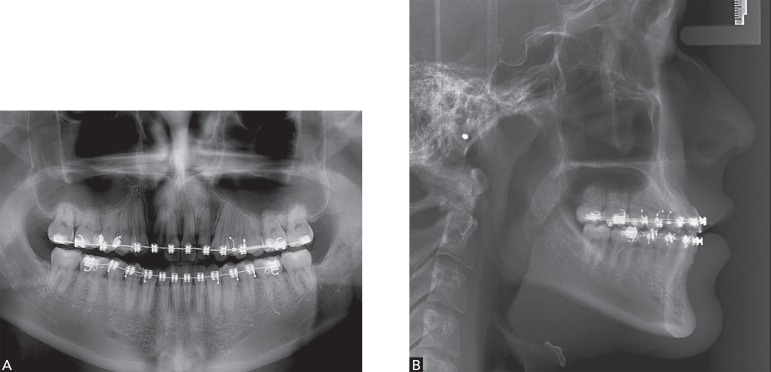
Radiographs at treatment completion: A) Panoramic radiograph, B) CephalometA B ric
